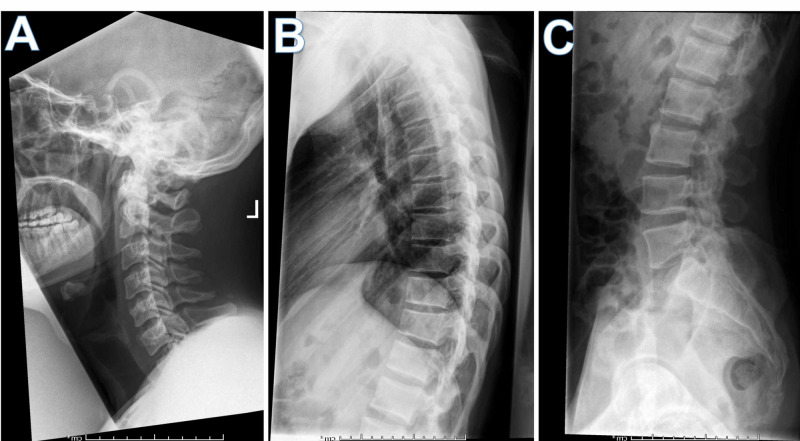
Lateral radiographs of the (A) cervical, (B) thoracic and (C) lumbar spine show no evidence of syndesmophytes, bamboo spine, or ossification of spinal ligaments, joints, or discs excluding ankylosing spondylitis
